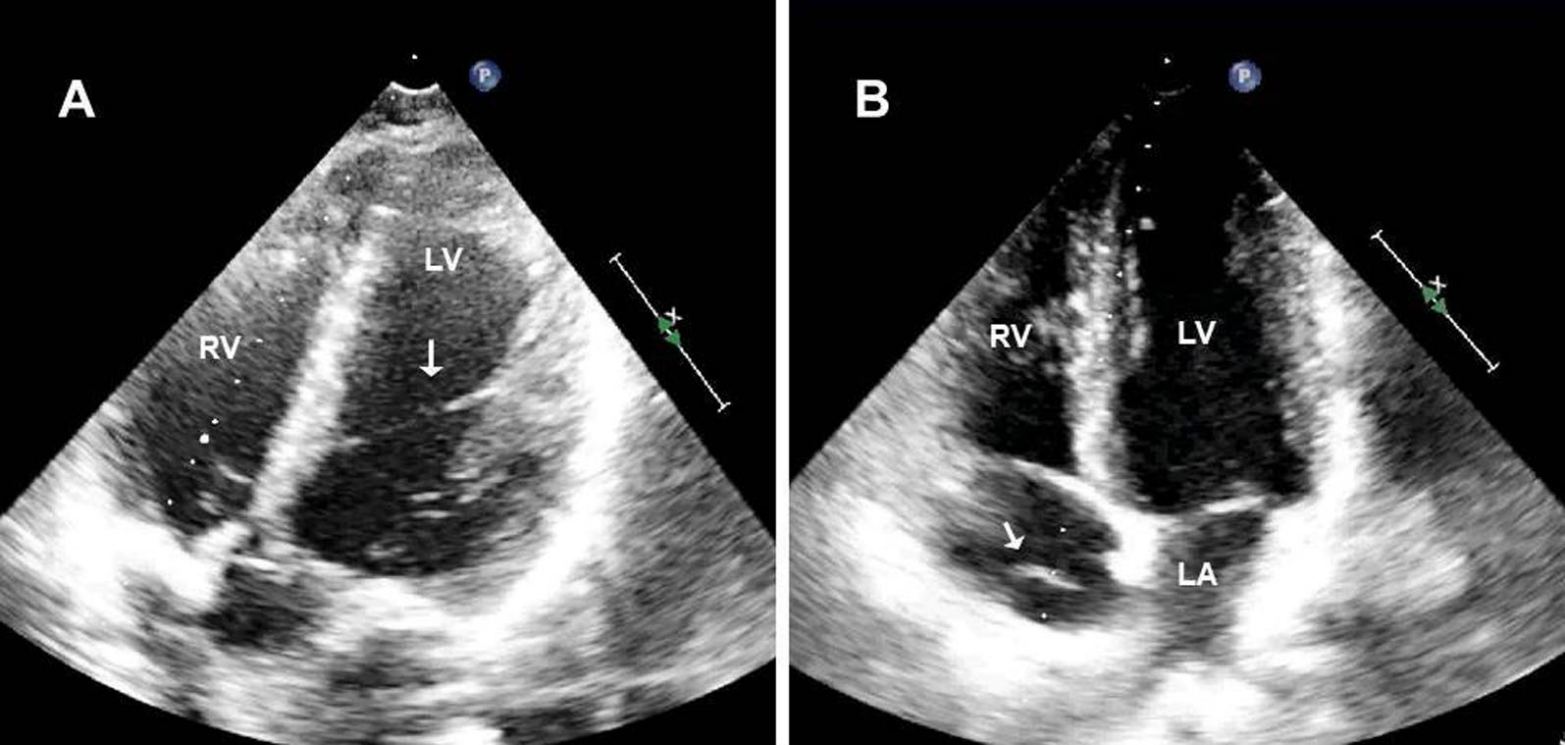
A Modified apical five-chamber imaging shows the left ventricular false tendon extending between the interventricular septum and the left ventricle lateral wall. B In the four-chamber imaging of the same patient, the Chiari’s network is observed in the right atrium
